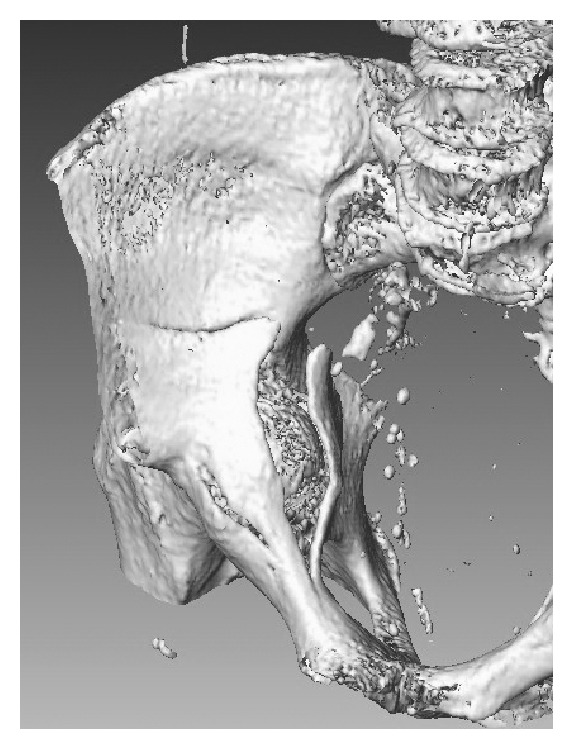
Intrapelvic 3D CT reconstructed view of the right Hip with protrusion of the right femoral head.
